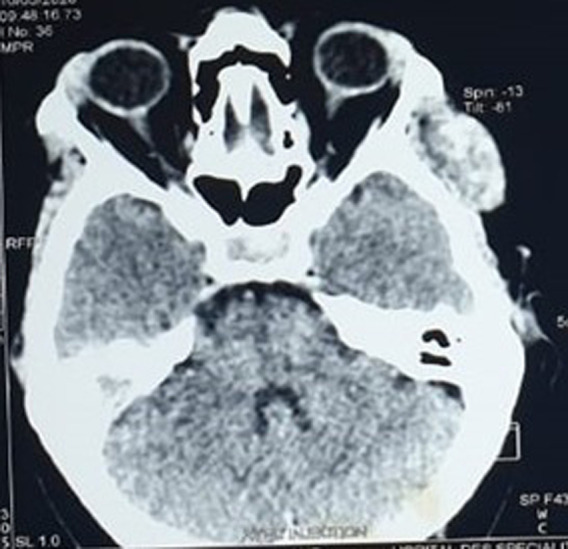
computed tomography scan on the axial plane shows a homogeneous contrast enhancement of the mass
